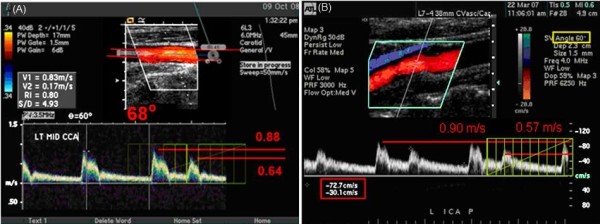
Arrhythmia Doppler Waveforms. Note the variability in systolic velocities with different preceding diastolic periods.
